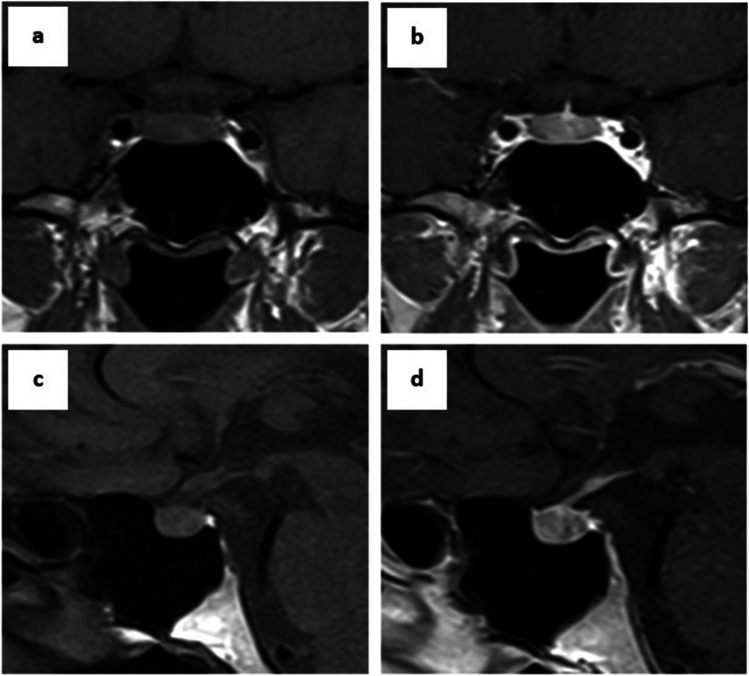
Frontal and sagittal MRI demonstrating a normal pituitary without signs of microadenoma in the presented patient: a Frontal T1-weighted pre-contrast scan. b Frontal T1-weighted post-contrast scan. c Sagittal T1-weighted pre-contrast scan. d Sagittal T1-weighted post-contrast scan
